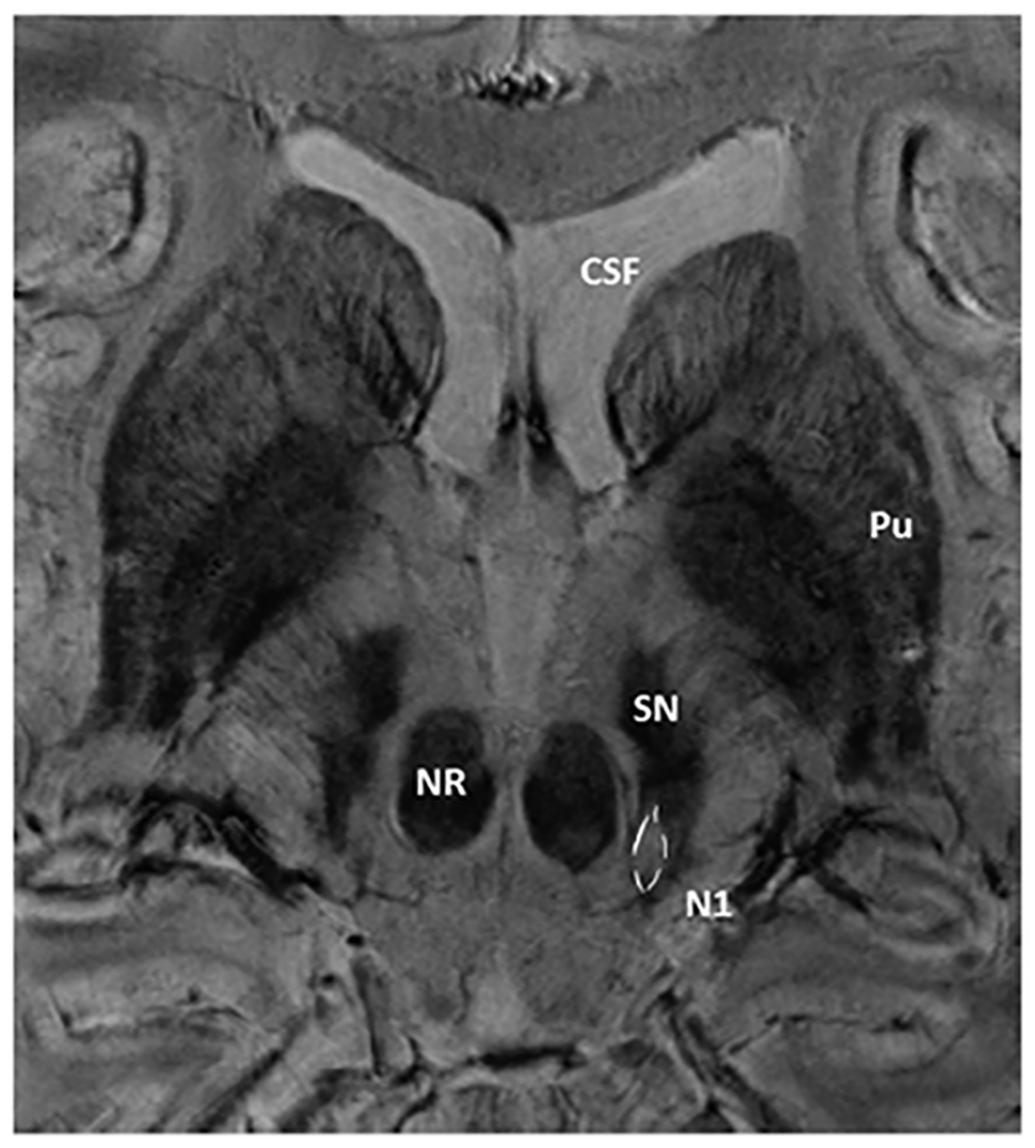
SWI 7T MRI of the midbrain and the surrounding structures. CSF: cerebrospinal fluid, Pu: putamen, SN: substantia nigra, NR: Nucleus ruber, N1: Nigrosome 1.
